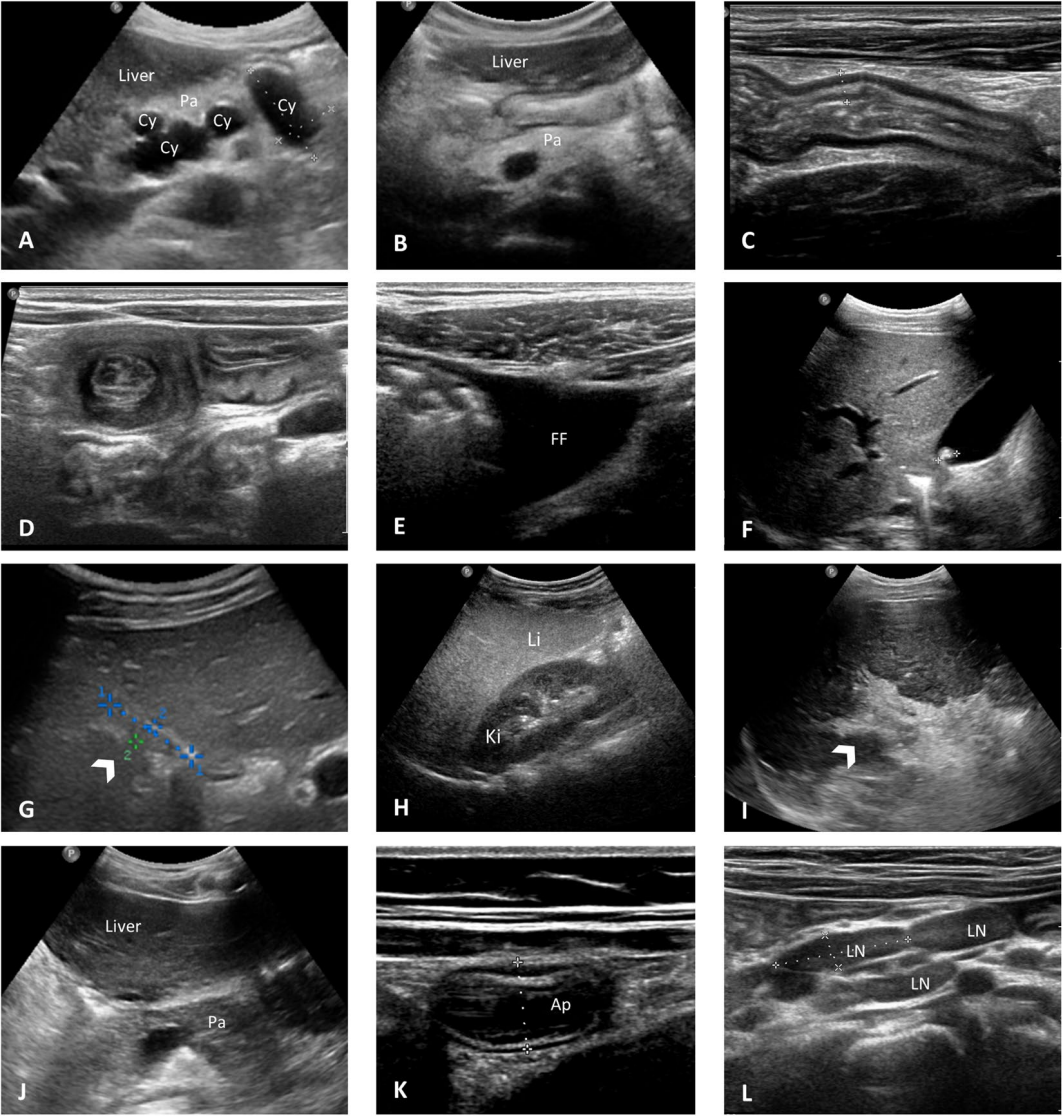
Specific US abnormalities identified in the Jena University CF cohort. (A) Pancreatic cystosis in an asymptomatic 41-year-old female patient heterozygous for *G551D* showing a hyperechoic pancreas (Pa) containing multiple cysts (Cy) with sizes approximately of 21 × 34 × 20 mm and 21 × 40 × 24 mm. CFAbd-Score: 82 (*range: highest burden of symptoms [0 points] to no symptoms [100 points], respectively). (B) Hyperechoic pancreas (Pa) with fat replacement as typical for pancreatic lipomatosis in a 10-year-old female patient homozygous for *F508del* with pancreatic insufficiency (symptom score: 95). (C) Longitudinal US image of the bowel wall in the terminal ileum measuring approximately 5 mm in a context of thickened bowel wall in a 19-year-old patient homozygous for *F508del* (symptom score: 81). (D) Transversal section of the small bowel showing the classic target sign appearance of intussusception in a 3-year-old female asymptomatic patient homozygous for *F508del* (symptom score: 96). (E) Free fluid (FF) in the right lower quadrant in a 32-year-old male patient homozygous for *F508del* with severe liver disease (symptom score: 80). (F) Single gallstone in a 12-year-old male asymptomatic patient heterozygous for *G551D* (symptom score: 100). (G) Gallbladder (arrow) in a 11-year-old female patient heterozygous for *G551D* measuring 18 × 4 mm in a context of micro-gallbladder (symptom score: 88). (H) US image of liver steatosis in a 4-year-old male patient homozygous for *F508del*, showing diffused increased echogenicity of the right lobe of the liver (Li) relative to right kidney cortex (Ki) (symptom score: 85). I) Severe periportal fibrosis (arrow) in a 28-year-old male patient heterozygous for *F508del/R347P w*ith cystic fibrosis liver disease (CFLD) (symptom score: 87). (J) Isoechoic pancreas (Pa) relative to liver in a 18 year-old female patient heterozygous for *G551D* with pancreatic sufficiency (PS) in longitudinal sonogram at level of the pancreatic head (symptom score: 99). (K) US scan of the appendix (Ap) in a 10-year-old asymptomatic female patient homozygous for *F508del*. Note the thickened aspect of the appendix (diameter of 9 mm) (symptom score: 95). (L) Enlarged mesenteric lymph nodes (LN) greater than 18 mm in the larger axis in a 10-year-old asymptomatic patient homozygous for *F508del*, in a context of an inflammatory etiology (symptom score: 95).
